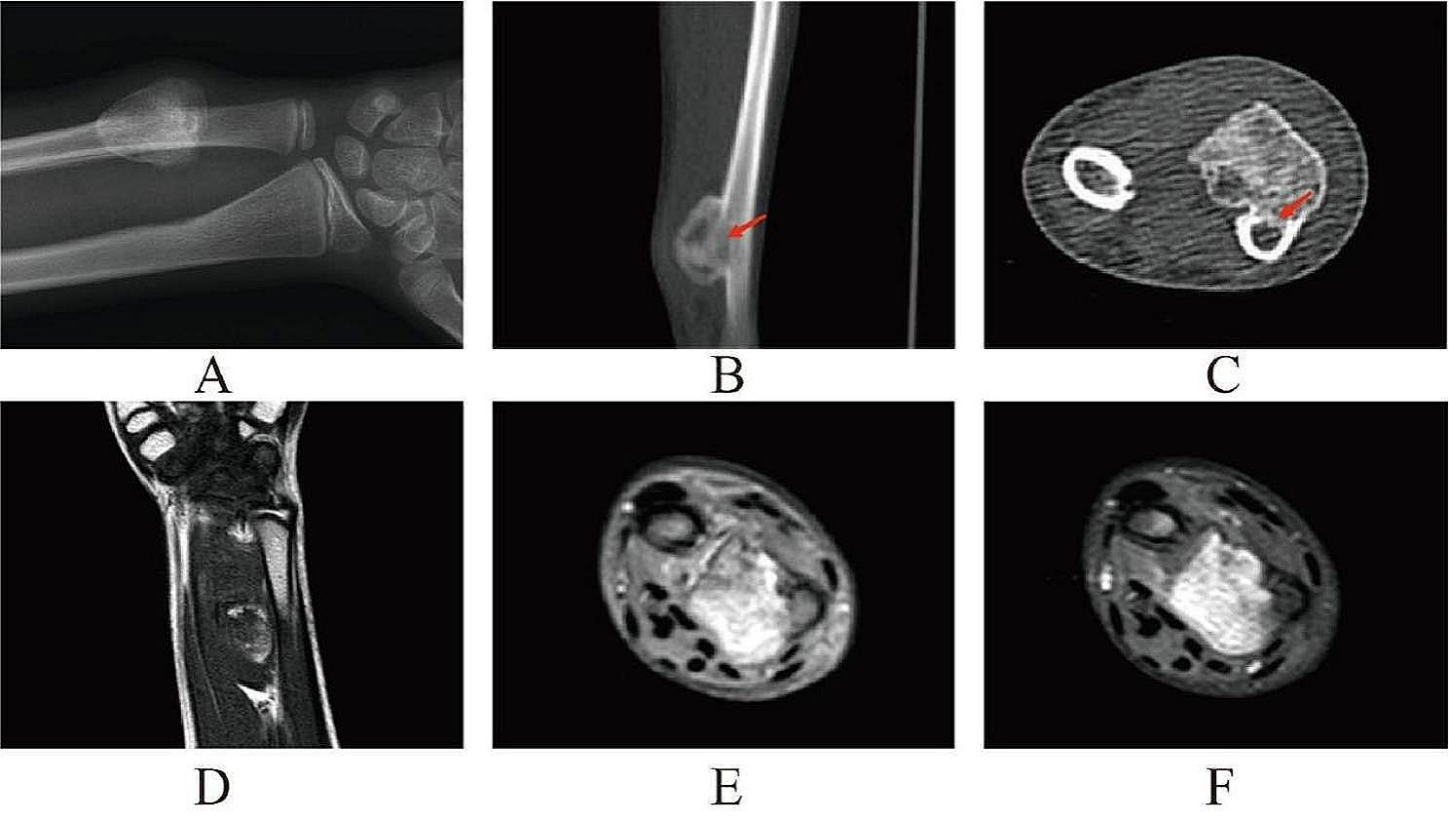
(A) A type of circular ossification was found in plain X-ray of the right ulna, which was closely related to the ulna. (B, C) The CT scan revealed that the lesion infiltrated the cortical bone of the ulna and communicated with the medullary cavity(arow). (D) On T1-weighted images showed inhomogeneous signal clumps adjacent to the ulnar and radius on the volar aspect of the right forearm. (E) On T2 with fat suppression, the lesion showed a predominantly high intensity signal. (F) On gadolinium-enhanced images showed inhomogeneous high signal throughout the tumor
